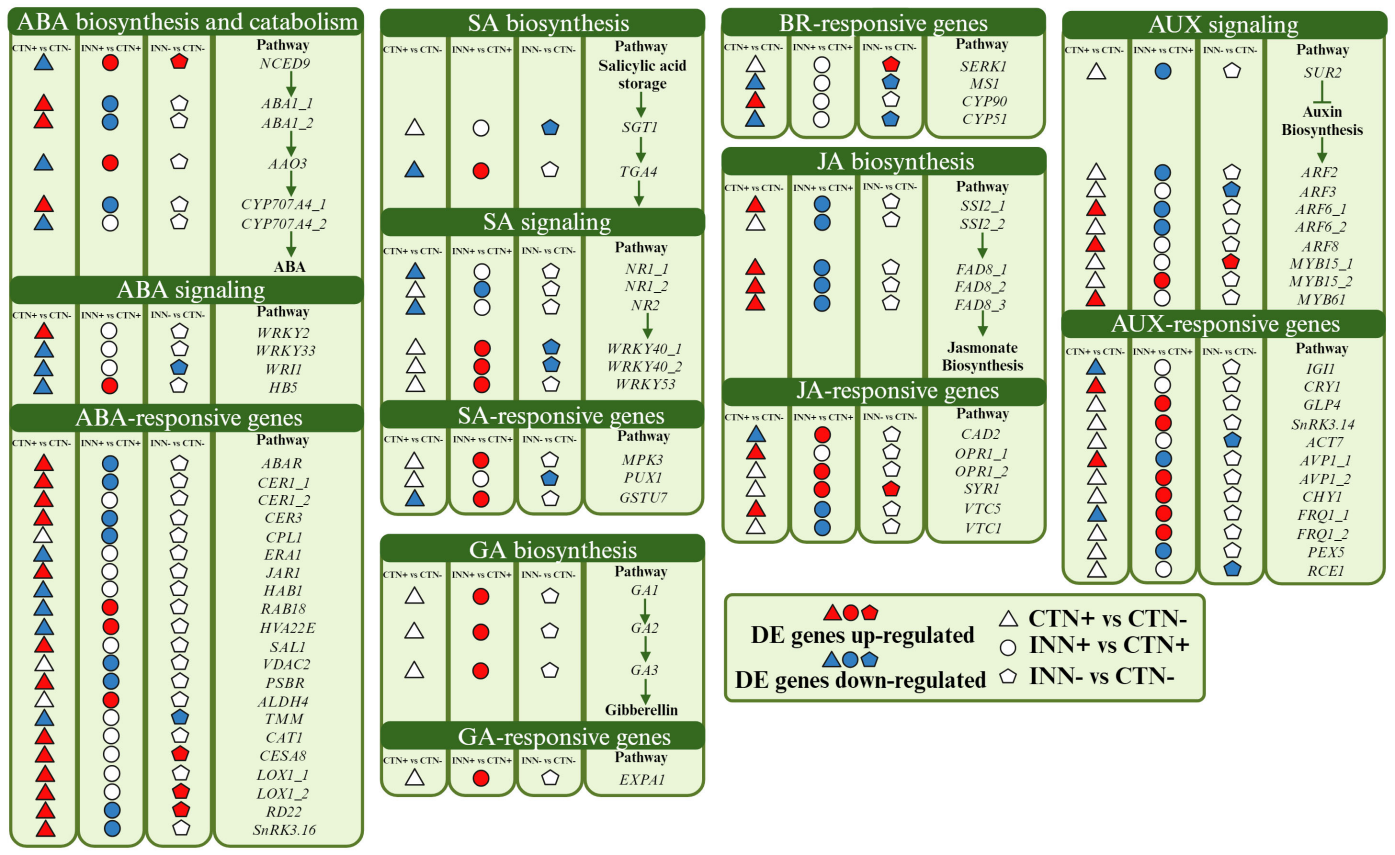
Overview of a scheme showing the regulation of hormone pathways. This model presents the differentially expressed genes of maize plants inoculated with *H. seropedicae* ZAE94 (IN) or mock-inoculated (CT) and cultivated in 0.3 mM (N-) or 3 mM (N+) of N. Up-regulated (red colors) or down-regulated (blue colors). White Symbol represents expressed genes, but not differentially regulated. ABA, abscisic acid; GA, gibberellin; AUX, auxin; JA, jasmonic acid; SA, salicylic acid; BR, brassinosteroid. Created with bioRender.com accessed on 01 December 2023.
